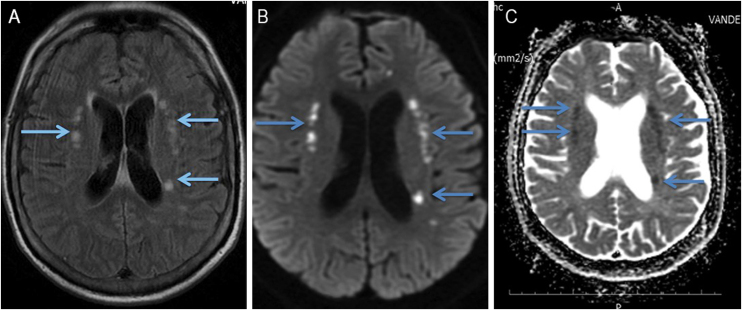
Magnetic resonance imaging of the brain. (a) FLAIR (fluid-attenuated inversion recovery) showing small foci of hyperintense signal in the periventricular and subcortical white matter of the bilateral frontal and parietal lobes. (b) DWI (diffusion-weighted imaging) showing hyperintense signal from the punctate foci seen on FLAIR (fluid-attenuated inversion recovery), suggesting cytotoxic edema. (c) ADC (apparent diffusion coefficient) MAP confirms the diffusion findings, showing restricted diffusion.
